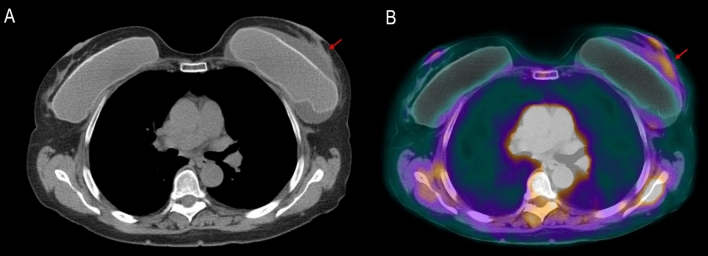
Axial unenhanced PET/CT image shows a small fluid collection surrounding the left breast implant (a, arrow) with mild tracer uptake on PET/CT fused image (b, arrow)
